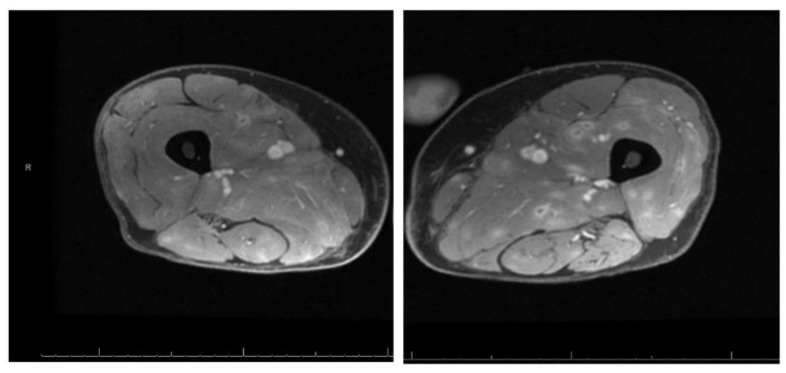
MRI of bilateral thighs with diffuse edema and multiple nodular and ring enhancing lesions.
